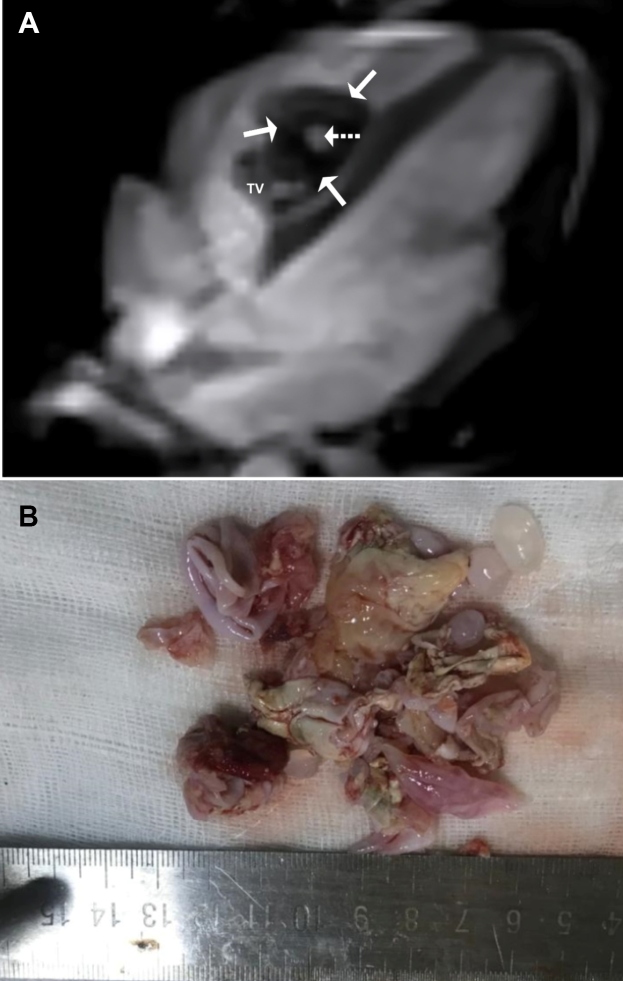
(A) Four-chamber steady-state free precession cine MR image. Hyperintense cystic areas (dashed arrow) can be seen in the hypointense mass (arrows) protruding from the interventricular septum into the right ventricle.(B) Cyst material surgically extracted from the lung and heart. TV: tricuspid valve.
